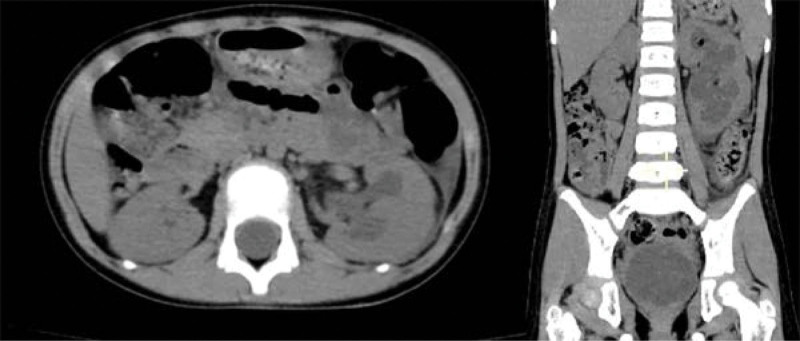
Sagittal and coronal view of postoperative lower abdominal CT (Case 3). CT = computed tomography.
